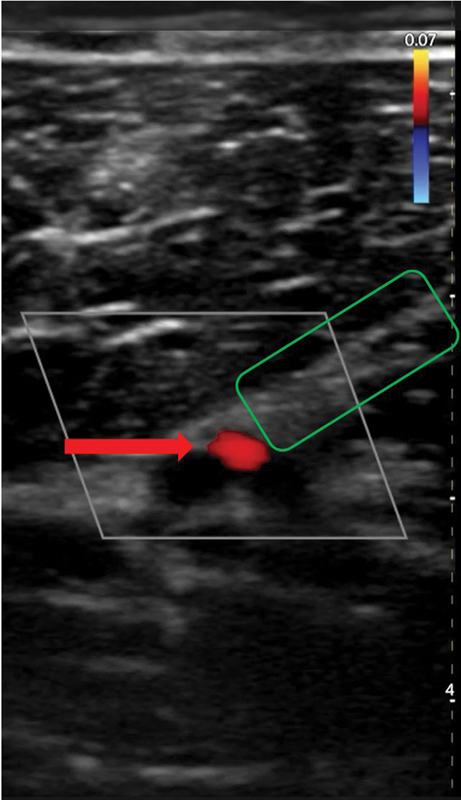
Color Doppler ultrasonography. A wireless handheld 12 MHz device was used. The red arrow shows the descending branch of the lateral femoral circumflex artery. The green box shows the septum dividing the vastus lateralis and the rectus femorismuscles.
